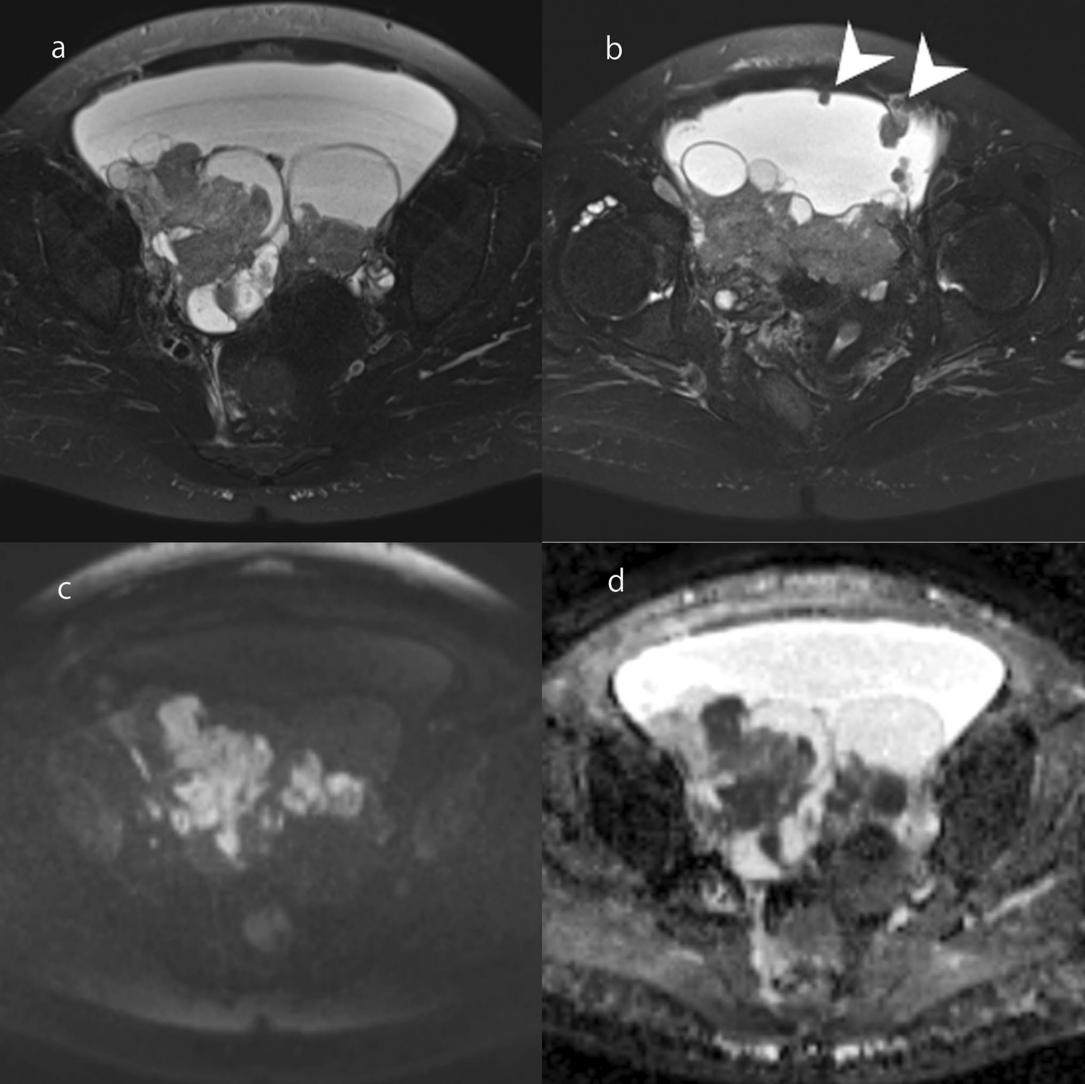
High-grade serous carcinoma. a FS-T2WI shows bilateral ovarian solid and cystic masses. Solid components show hypointensity compared with serous borderline tumor. A large amount of ascites is visible. b FS-T2WI shows some peritoneal dissemination (arrowhead). c The solid components are markedly hyperintense on DWI (high *b* value). d The solid components are markedly hypointense on ADC maps
